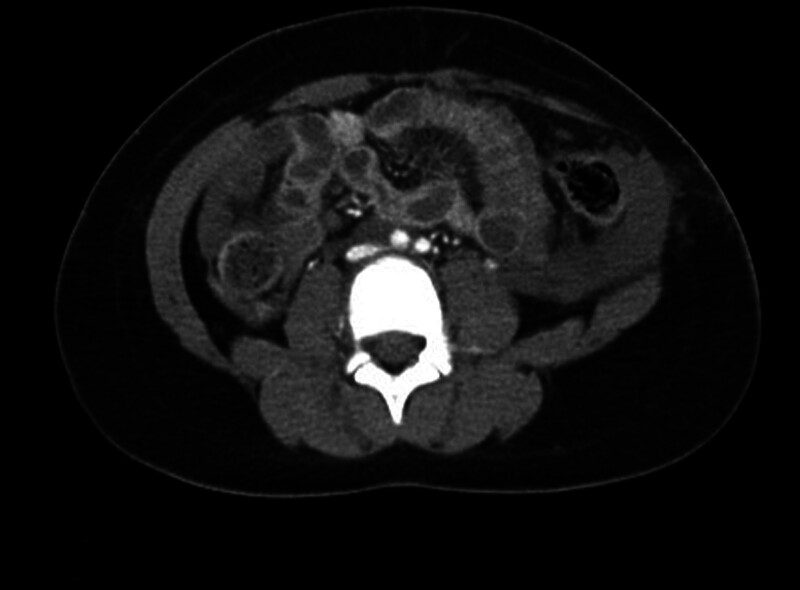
Initial abdominal computed tomography (CT) showing bowel herniation through the muscular defect in the left lateral abdominal wall. A small amount of fluid around the liver and spleen, mild thickening of the small bowel wall, and infiltration in the small bowel mesentery are also observed, indicating the possibility of small bowel injury.
